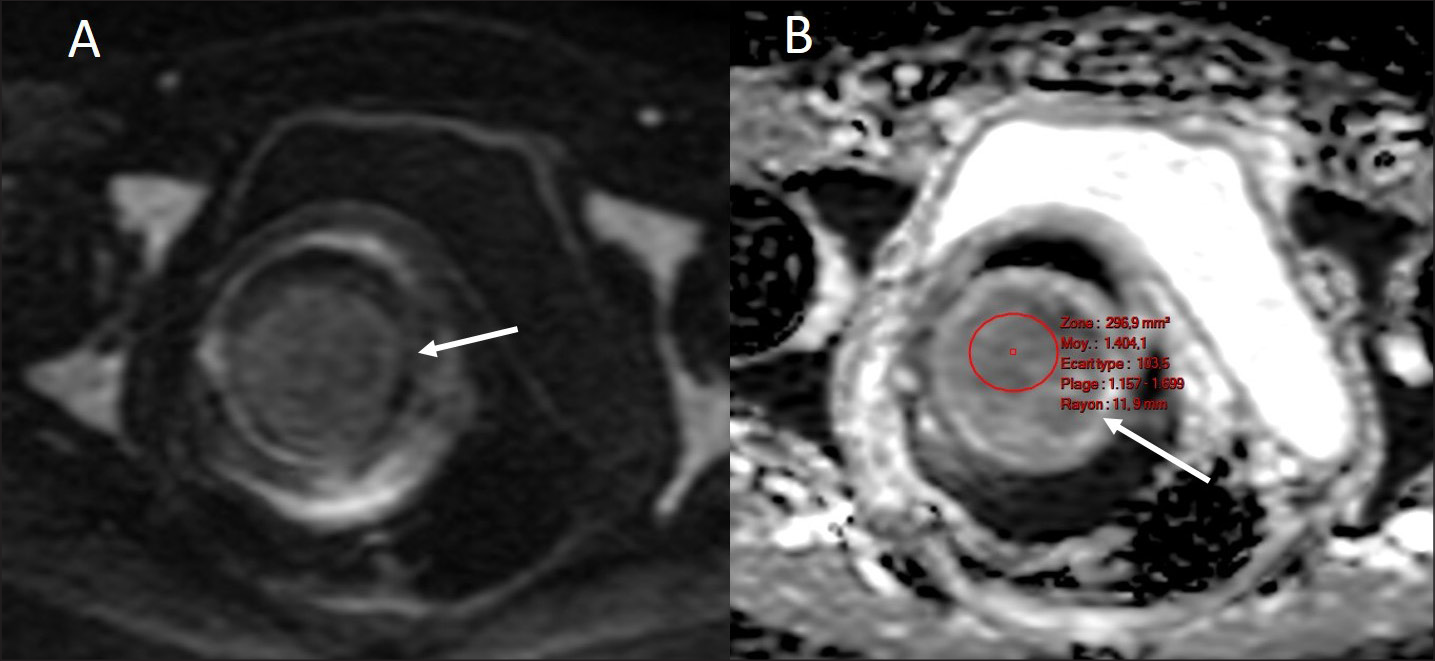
Sagittal gadolinium-enhanced fat-saturated T1-weighted image shows similar enhancement of the solid components of the mass and the myometrial tissue (Figure 3A). Sagittal gadolinium-enhanced fat-saturated T1-weighted image shows a very similar appearance between the solid component of the mass and myometrial tissue (Figure 3A, white arrow). This is connected to the endometrial cavity by a stalk (Figure 3A, 3B white head arrow).
